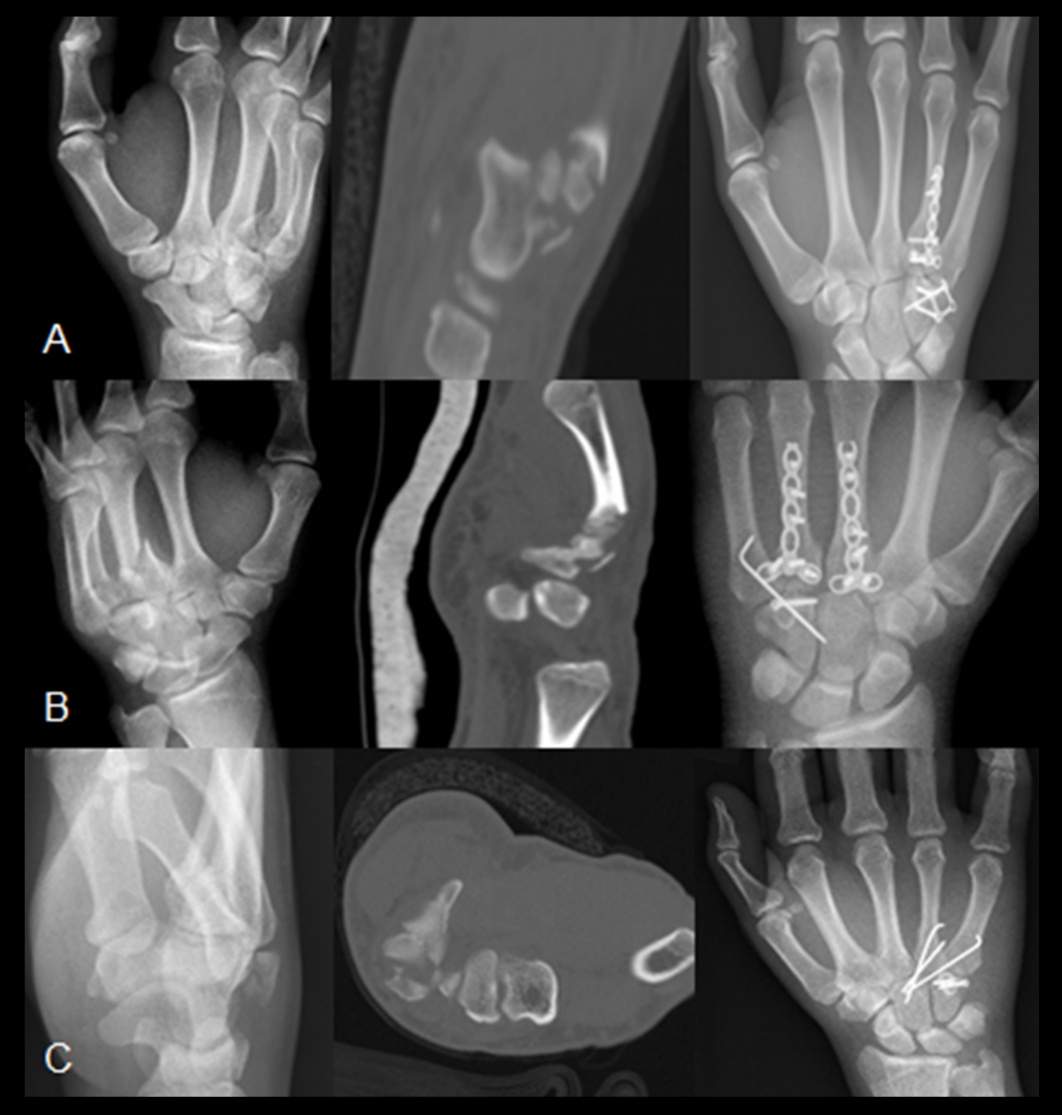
Three patients with coronal hamate’s fracture (A – grid plate OS, B – K-wire and screw OS, C – screw OS). From left to right: preoperative x-ray, preoperative CT scan, postoperative x-ray
